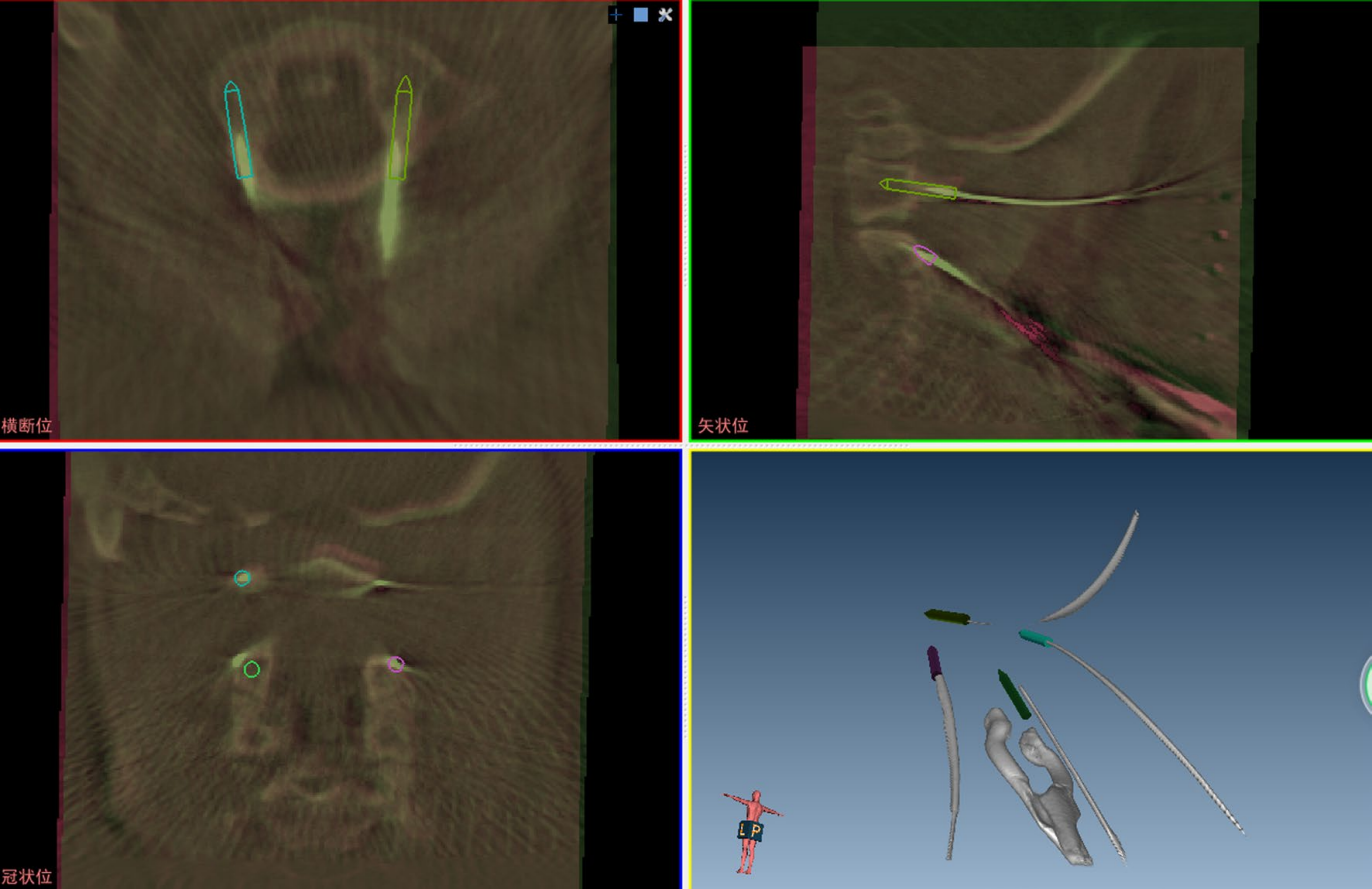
Representative fused images. Based on the manual fusion method, the screw planning image was matched with the guiding pin placement CBCT image via overlay technology in the axial plane, sagittal plane and coronal plane
